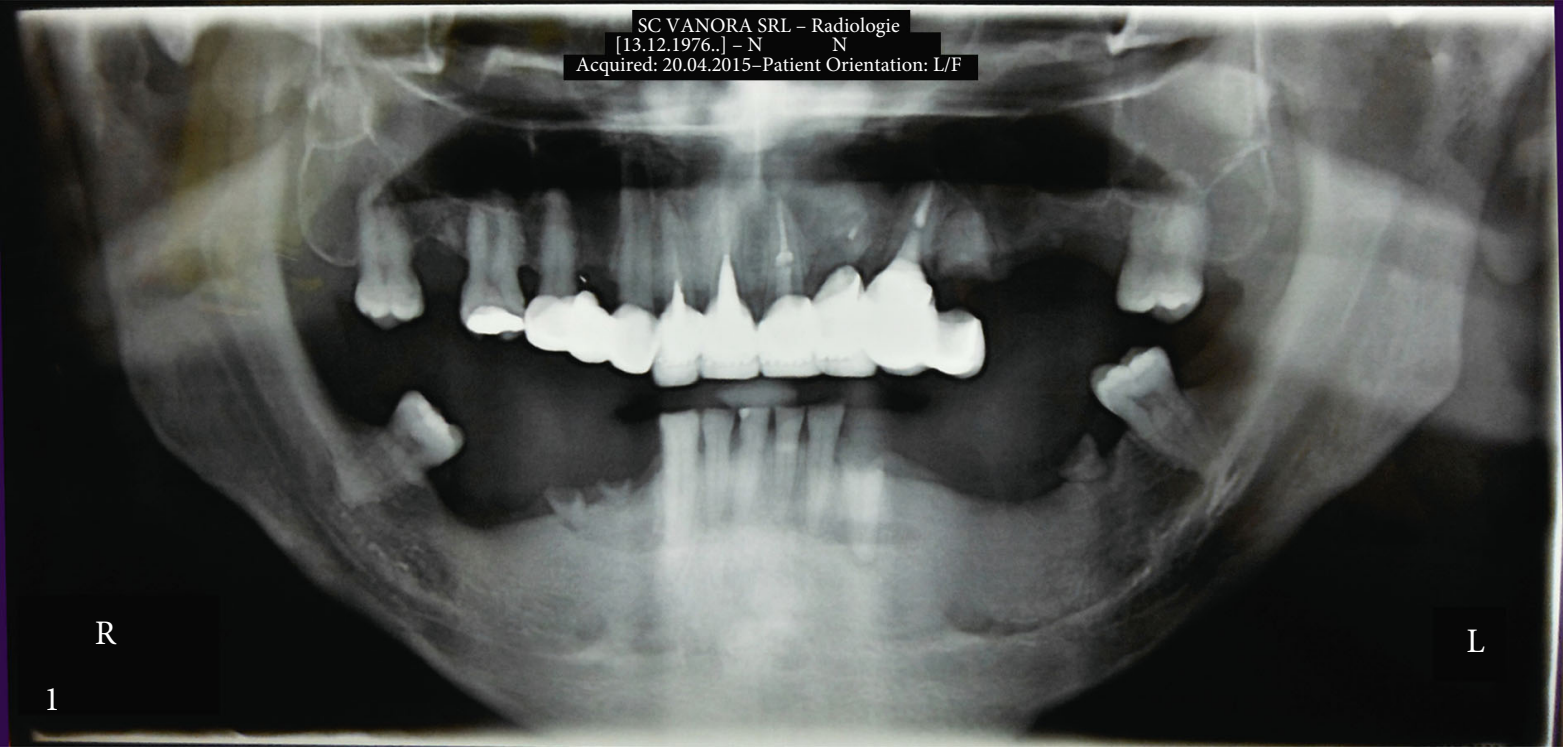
Initial orthopantomography.
